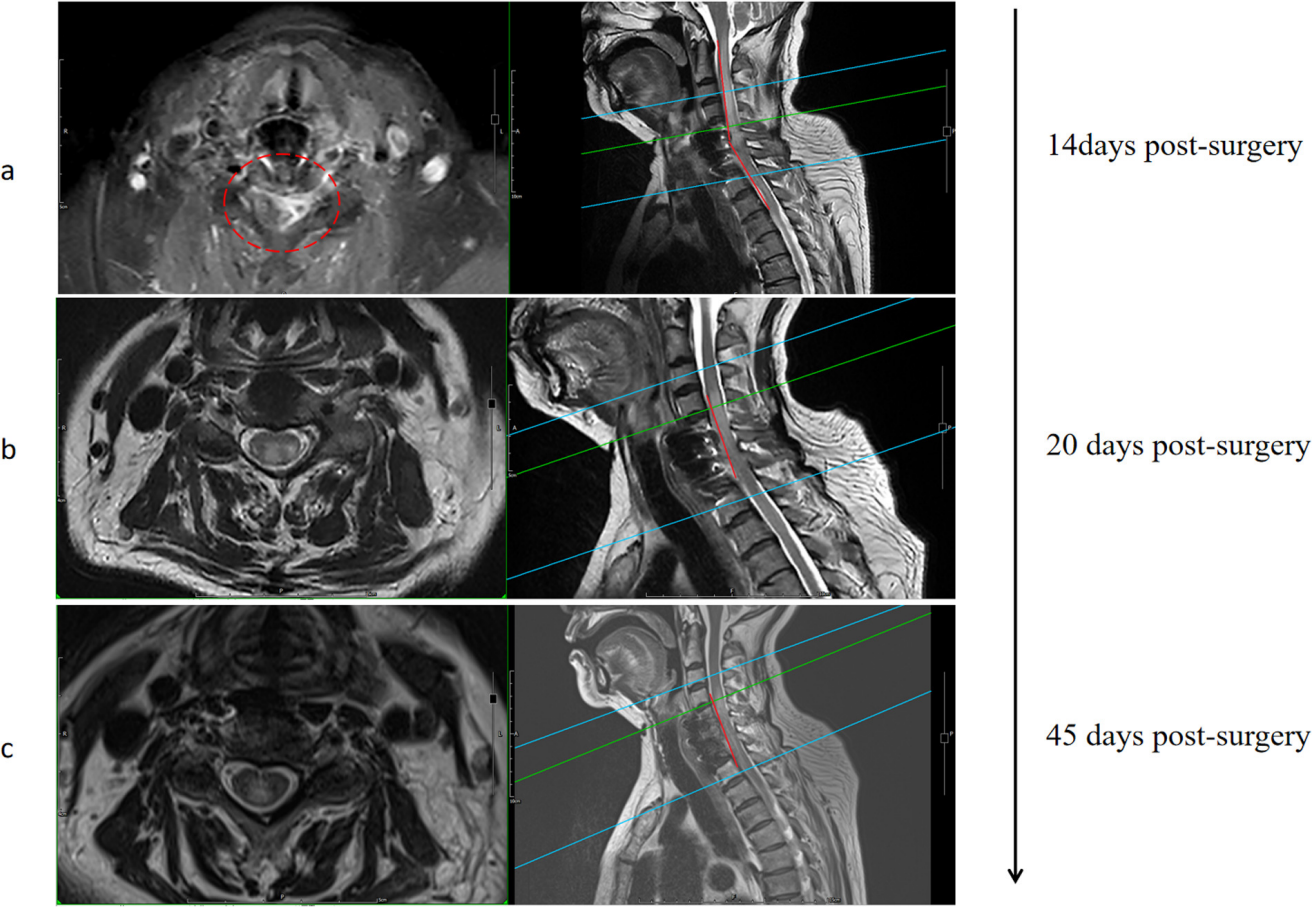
MRI imaging findings at different periods after the occurrence of axial pain after surgery (a) Imaging follow-up after the first empirical use of antibiotics (b) Imaging follow-up after antibiotic administration for one week after metagenomics revealed the causative organism (c) Imaging follow-up about 3 weeks after discharge.
